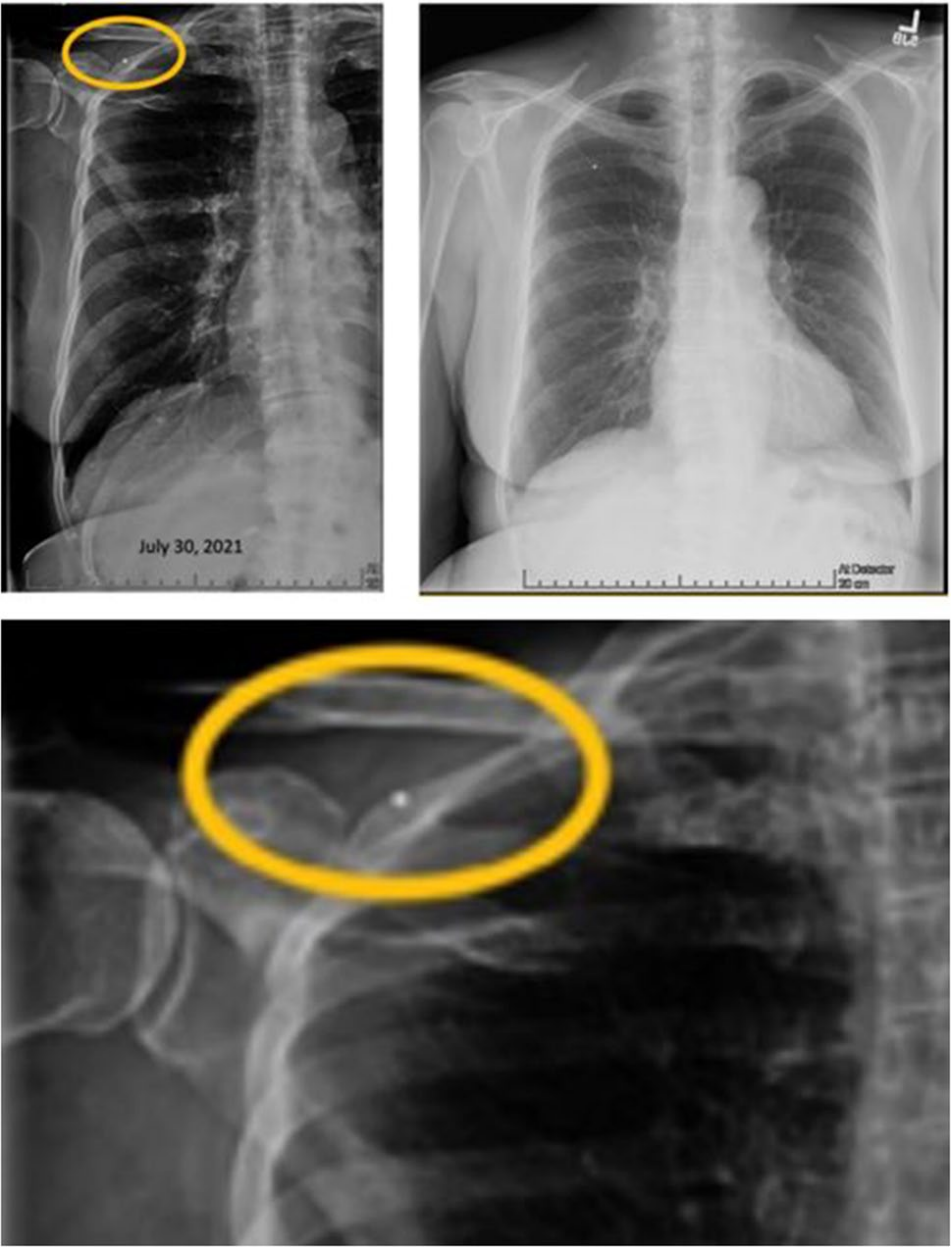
AP chest radiograph and higher coned down view of AP chest radiograph revealed a subtle right upper chest wall soft tissue density (yellow circle) indicated by the metallic marker denoting a palpable mass. Note the absence of rib destruction, rendering this lesion difficult to discern at all
